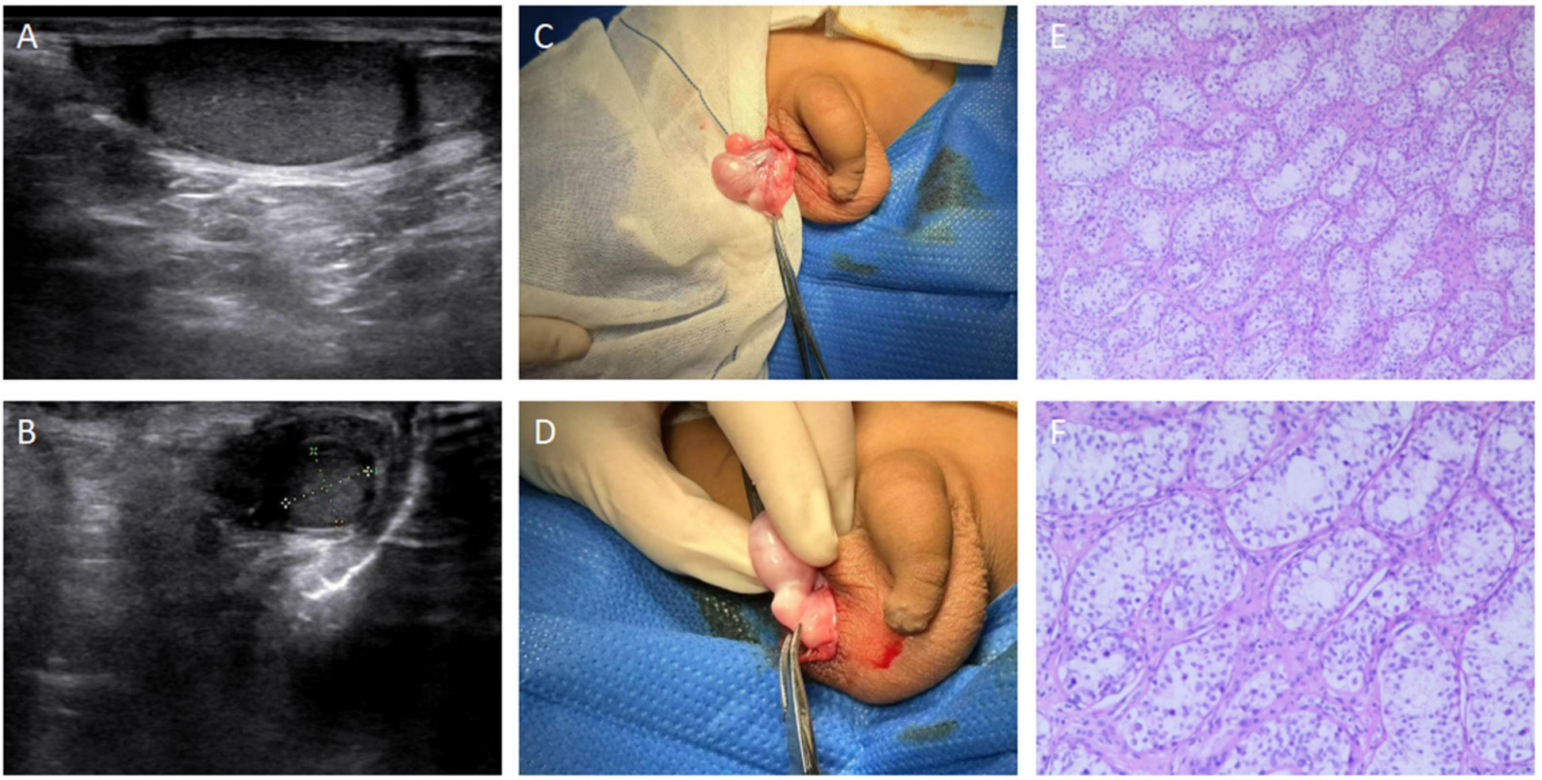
Preoperative Ultrasound, Intraoperative Photos, and Postoperative Pathology of Polyorchidism in a Pediatric Patient. (A) Preoperative ultrasound image showing a hypoechoic mass below the right testicle, measuring 0.6 × 0.5 cm. The echogenicity of the mass is similar to that of the testis, with clear borders and regular shape. The longitudinal axis of the right testis is 1.8 cm, and the transverse diameter is 1.0 cm. (B) Preoperative ultrasound image showing an additional right testis with a longitudinal axis of 0.9 cm and a transverse diameter of 0.6 cm. (C,D) Intraoperative photos showing the location of the additional testis. (E) Postoperative pathological examination showing convoluted seminiferous tubules (HE ×100). (F) Postoperative pathological examination showing convoluted seminiferous tubules (HE ×200).
